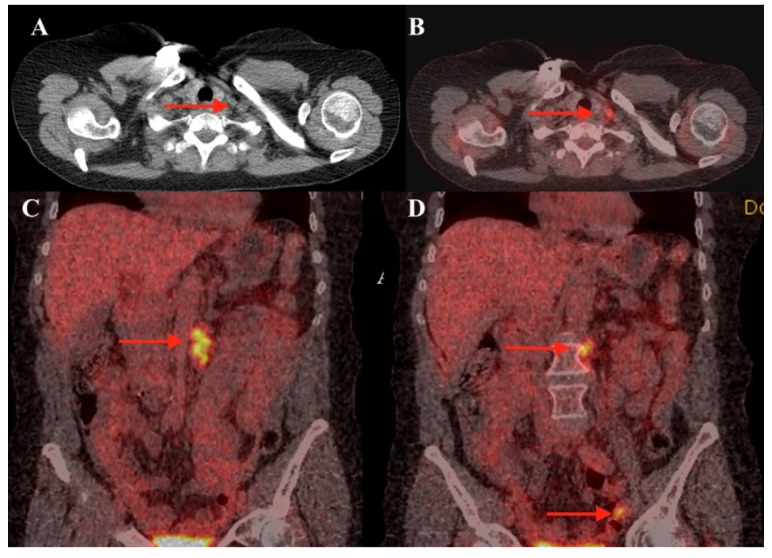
(A) F18-fluorodeoxyglucose positron emission tomography/computed tomography (F18-FDG PET/CT) axial CT section cervical level; (B) fused PET/CT image axial section at cervical level; (C) coronal section PET/CT image at abdominal level; and (D) coronal section PET/CT image pelvic level. Red arrows are showing high tracer uptake in the left supraclavicular lymph node, in the left latero-aortic lymph node and the left iliac extern pelvic lymph node.
